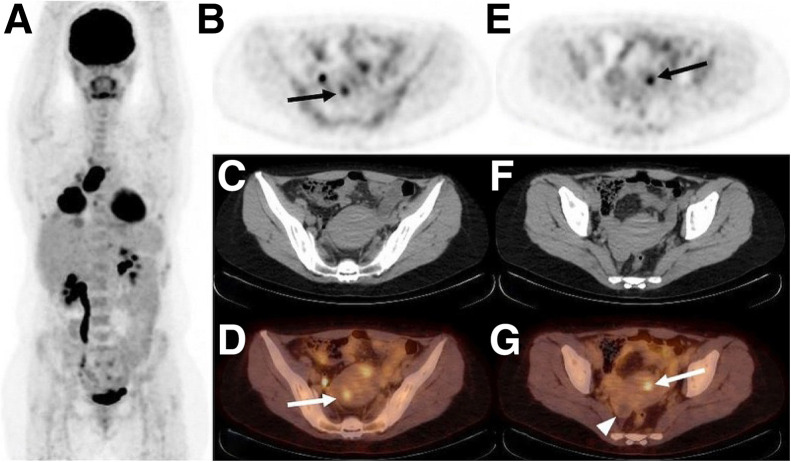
[^18^F]FDG PET/CT of 47-y-old woman with sarcomatous lung cancer and 11-y history of endometriosis at time of imaging. (A) Intense [^18^F]FDG uptake can be seen in primary lung cancer lesions as well as lymph node and pleural metastases. (B–G) Two foci of increased [^18^F]FDG avidity can be seen in endometriosis lesions in uterine wall via PET (B and E) and PET/CT (D and G) (arrows) but are not clearly visualized by CT alone (C and F). No [^18^F]FDG uptake was observed in known right ovarian endometrioma (arrowhead). (Reprinted with permission of (17).)
